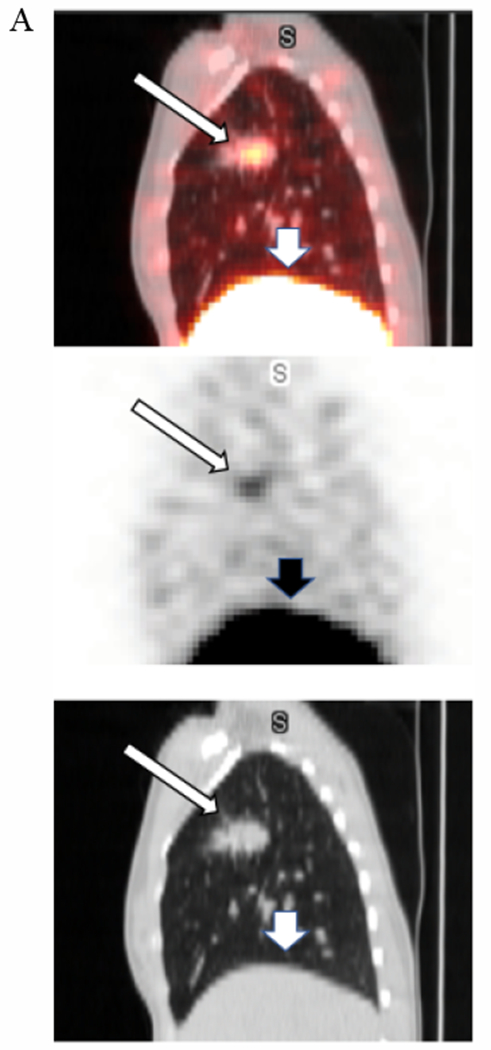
False positive. A 35-year-old female with NSCLC enrolled onto our study as HER2(−) based on pericardial effusion biopsy (HER2 2+; FISH ratio 1.22). The right upper lung lesion (the Target Lesion) is easily visualized (narrow arrows) on these fused SPECT/CT, SPECT, and low attenuation CT sagittal projections from the 71h post iv infusion of 128.4MBq[160.4ug] ^111^In-CHX-A”-DTPA trastuzumab imaging session. Tumor to Background Ratio is 6.7. The wider arrows show the liver.
